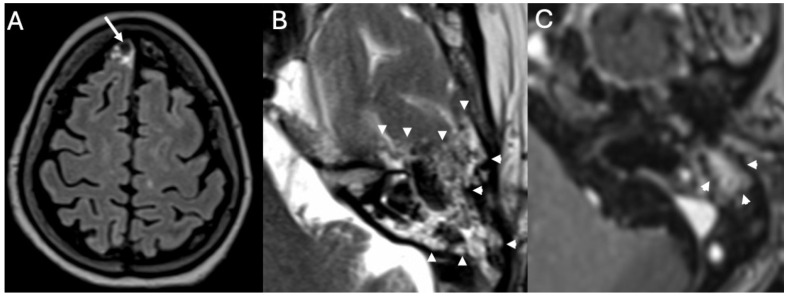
Meningitis due to *Streptococcus pneumoniae* in a 77-year-old patient. Axial enhanced FLAIR (A), T2 (B), and enhanced T1 (C) show leptomeningeal enhancement in the superior frontal region (arrow in (A)). There is acute mastoitidis on the left side (arrowheads in (B)) with patchy contrast enhancement (arrowheads in (C)).
